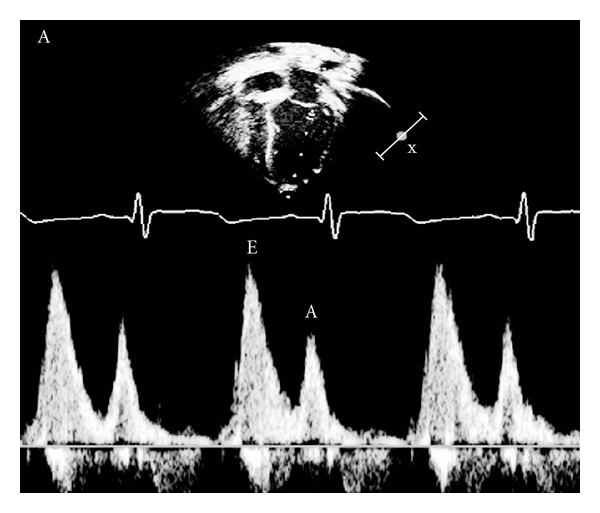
Pulsed-wave Doppler pattern of mitral inflow. It shows the peak velocities during early diastole (E) and atrial contraction (A).
